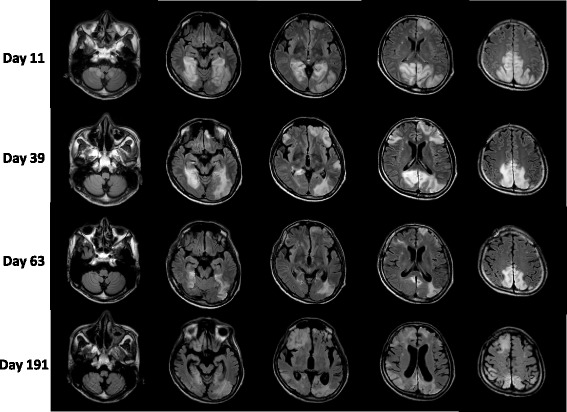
FLAIR images at days 11, 39, 63 and 191 show high intensity signals appearing recurrently in various cortical and subcortical areas, mainly in the bilateral occipital lobe, and that atrophic changes develop progressively over approximately 6 months
